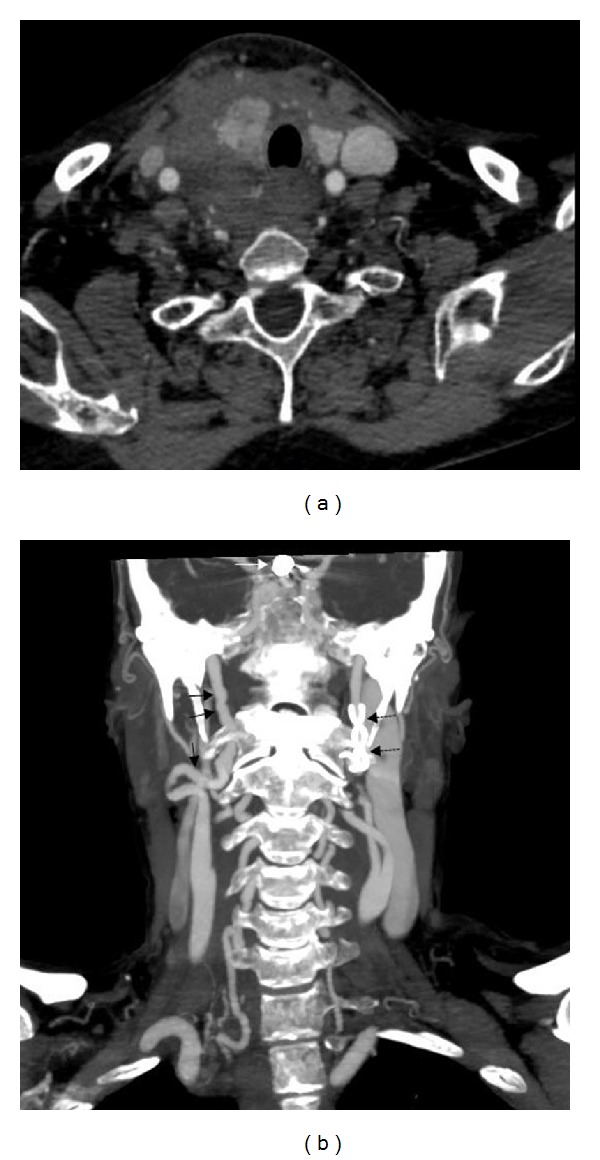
Contrast-enhanced angiographic CT (CTA). (a) Contrast-enhanced CT angiography in axial view shows the large right cervical hematoma causing anterior displacement of the right thyroid lobe and slight tracheal deviation to the left. (b) CT angiography in coronal maximal intensity projection (MIP) reformation showing the classical “strings of beads” sign in the right ICA (black arrows), typical for FMD, the stent in the left ICA (black dashed arrows), and the coil in the paraophthalmic segment of the right ICA (black arrow). No active bleeding is seen.
